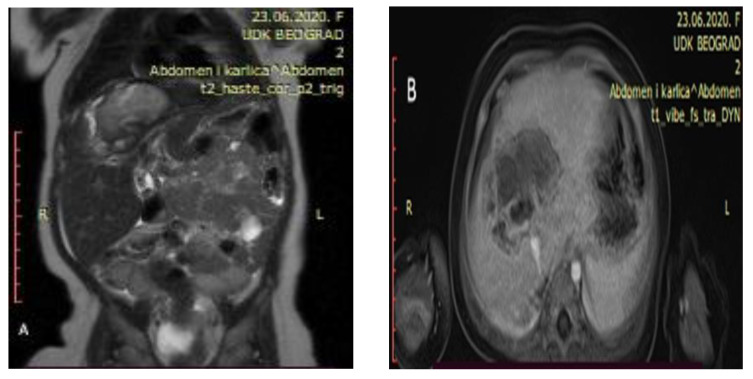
Control abdominal MRI after six cycles of chemotherapy showing a significant decrease in the tumor mass ((A) showing the coronal plane view of the MRI; (B) showing the axial plane view of the MRI).
